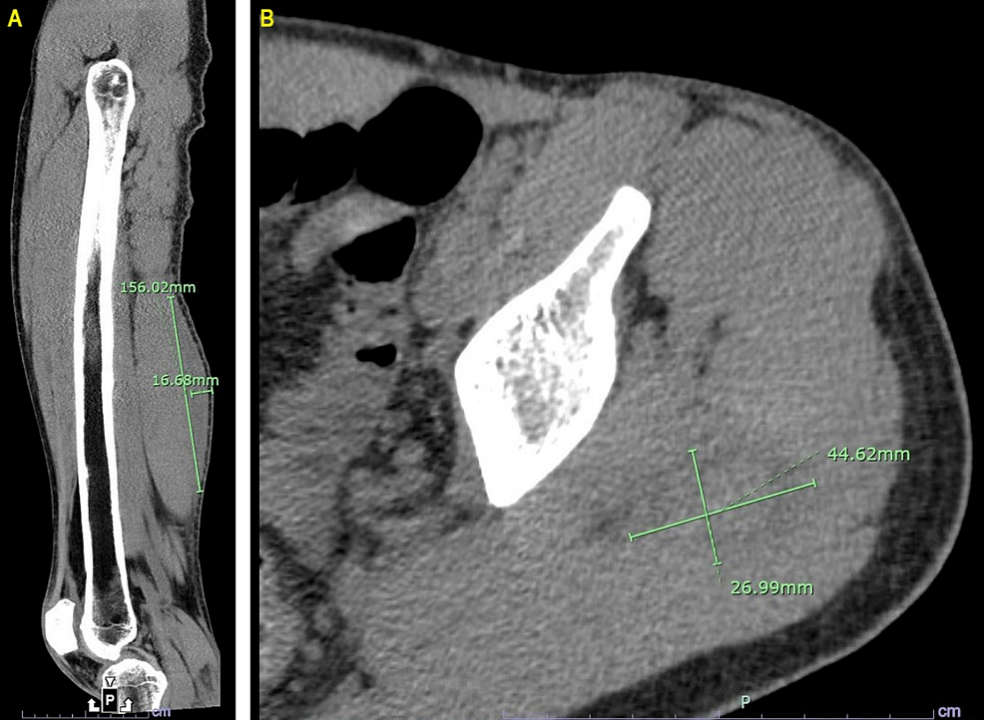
Computed tomography scan Computed tomography scan showing hematomas in the left posterior thigh musculature (A) and gluteus maximus (B).
